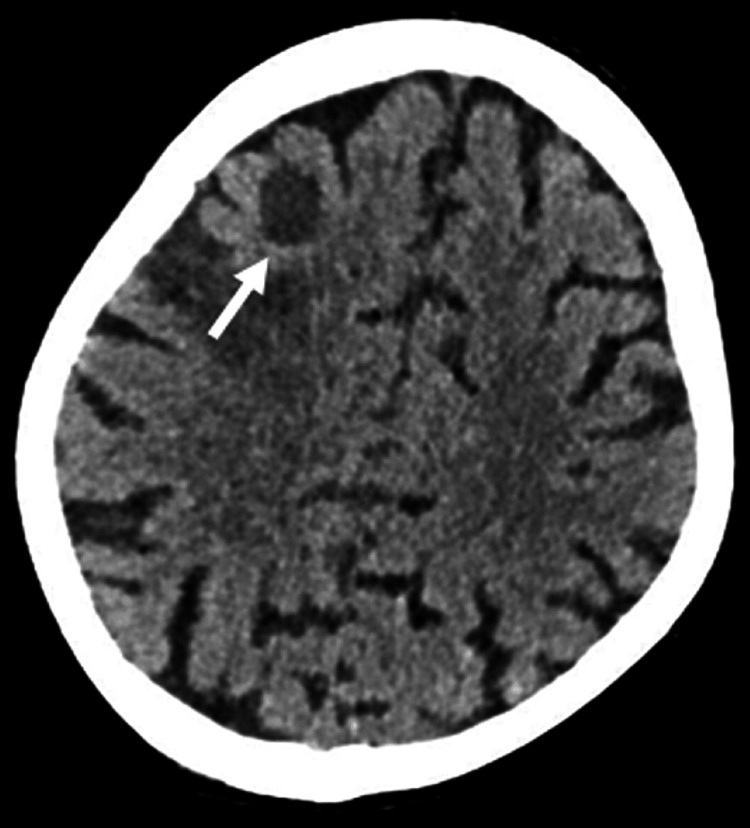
Non-contrast-enhanced CT (CECT) scan of the brain showing brain metastasis
